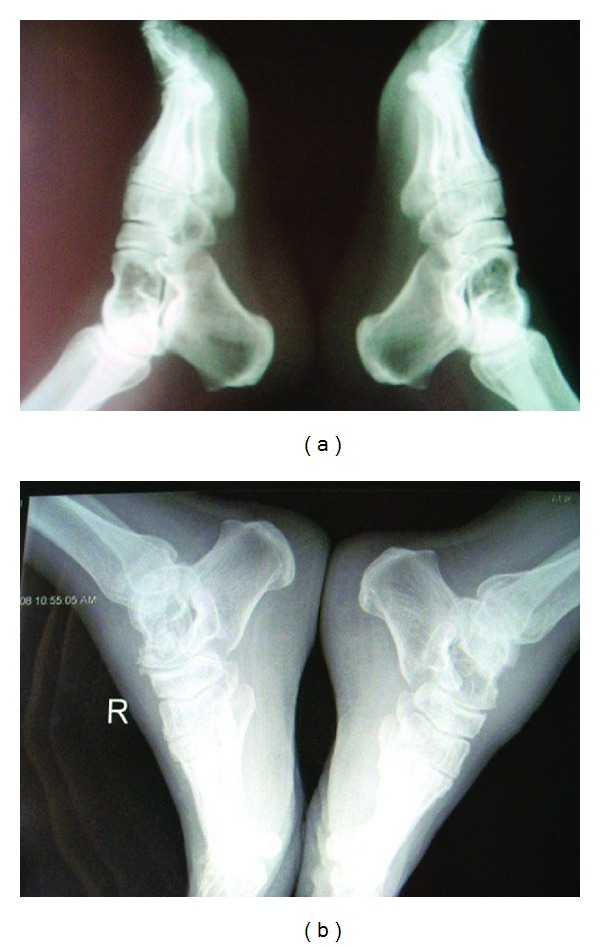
(a) Lateral radiographs of bilateral ankles showed multiple lytic lesions with sclerotic rims in both talus. (b) Lateral radiographs of bilateral ankles showed multiple lytic lesions with sclerotic rims in both talus.
